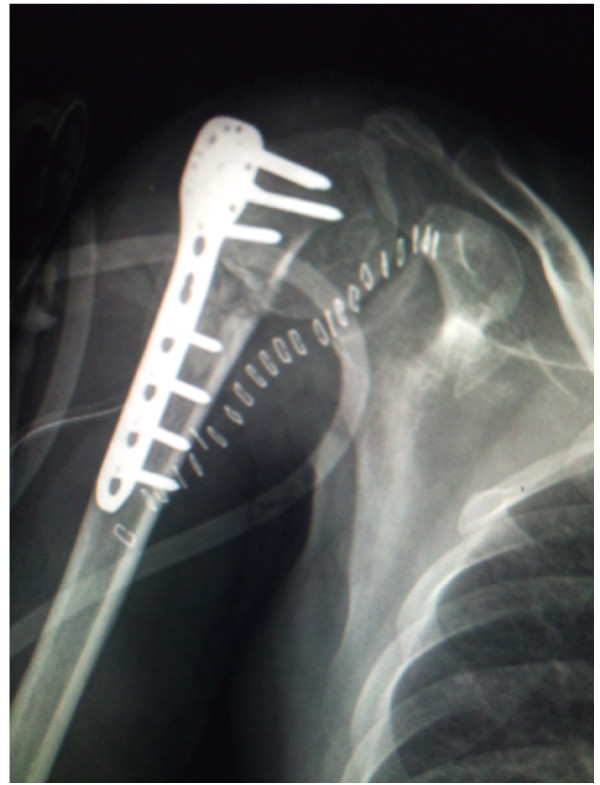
Immediate post operative X-ray in a patient showing varus collapse and plate pull out.
